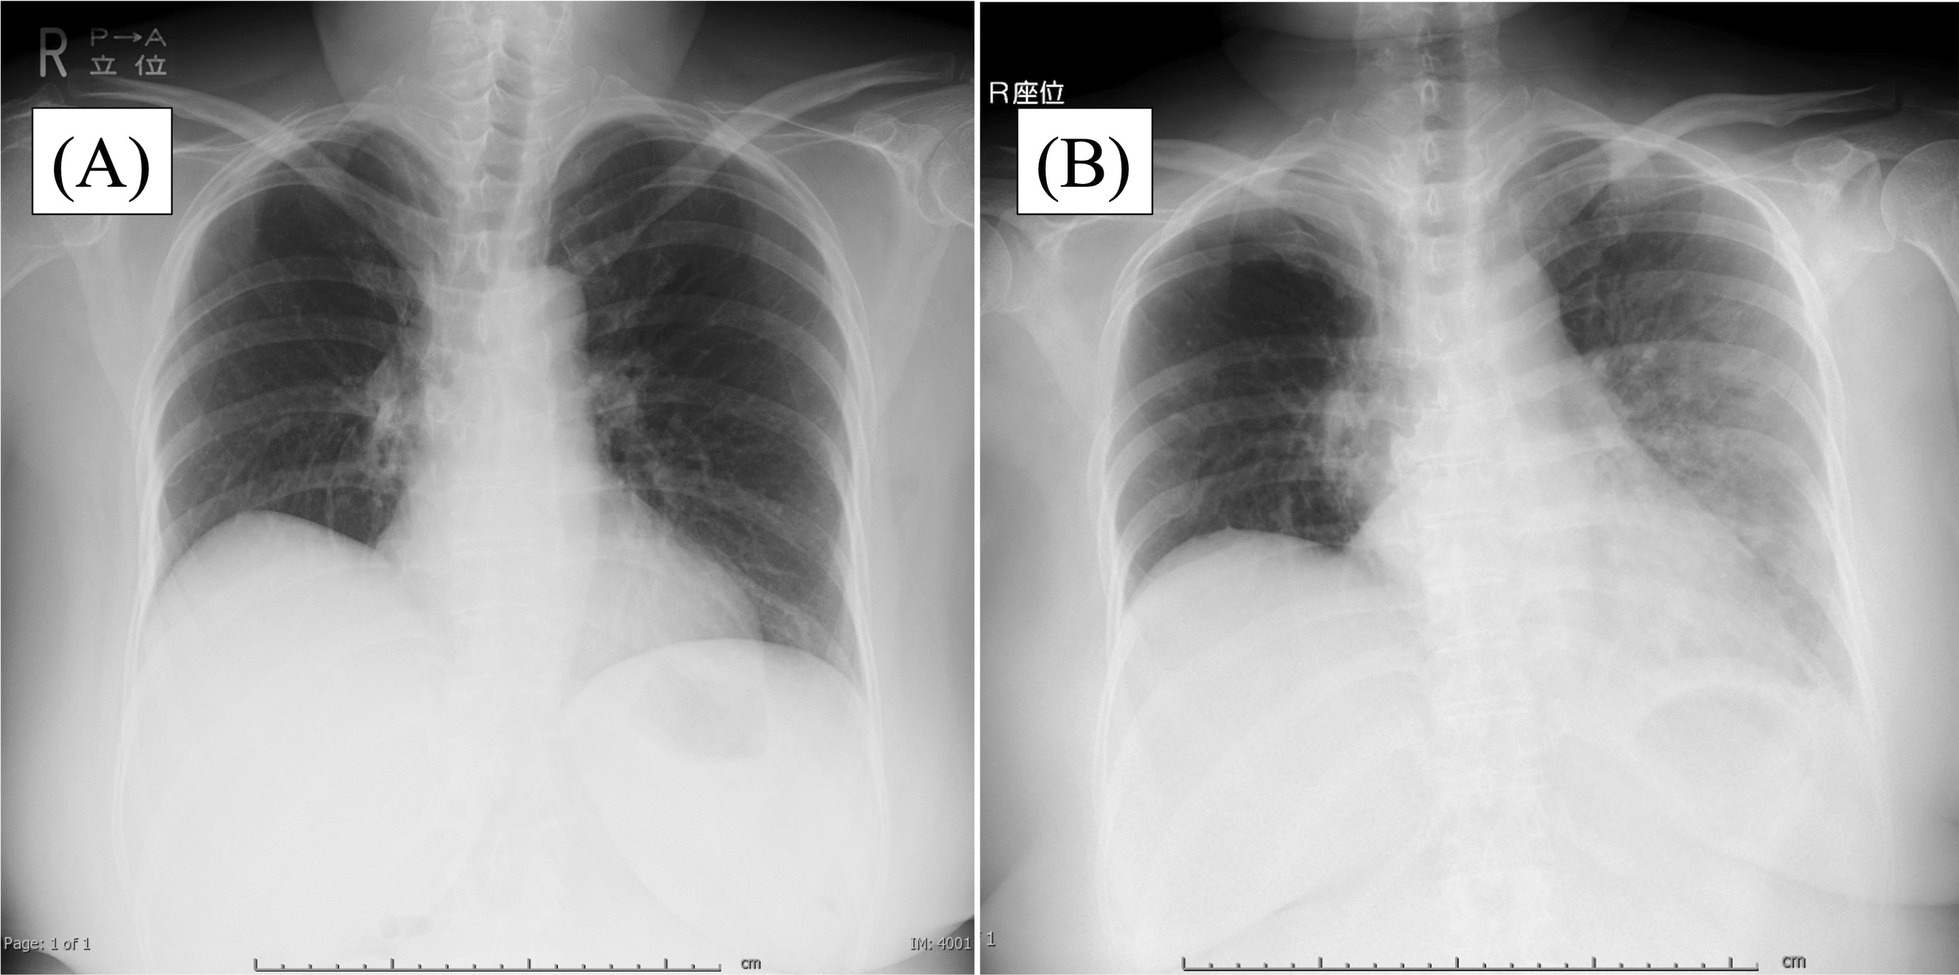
Chest radiograph at admission revealing no decreased permeability of the lung fields (a day 13). Chest radiograph at the onset of myocarditis revealed decreased permeability of the lung fields (b day 17)
